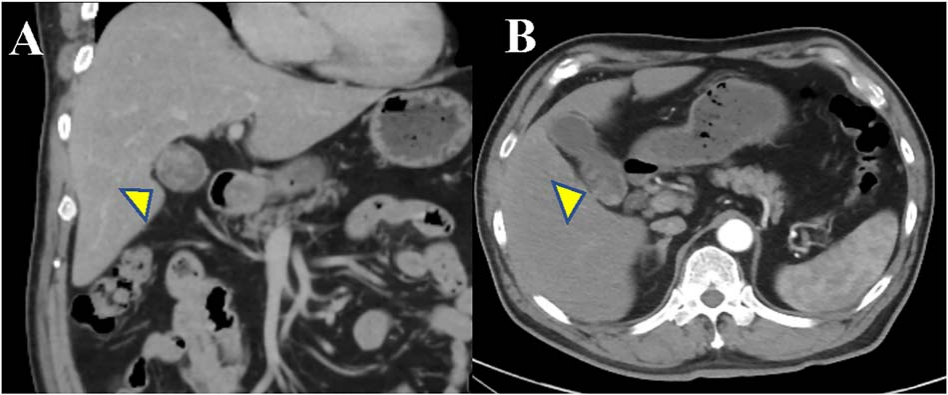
Abdominal CT findings. (A, B) The arterial phase shows a slightly enhanced low-density tumor, maximum of 27 mm in size, extending from the body to the bottom of the gallbladder.
